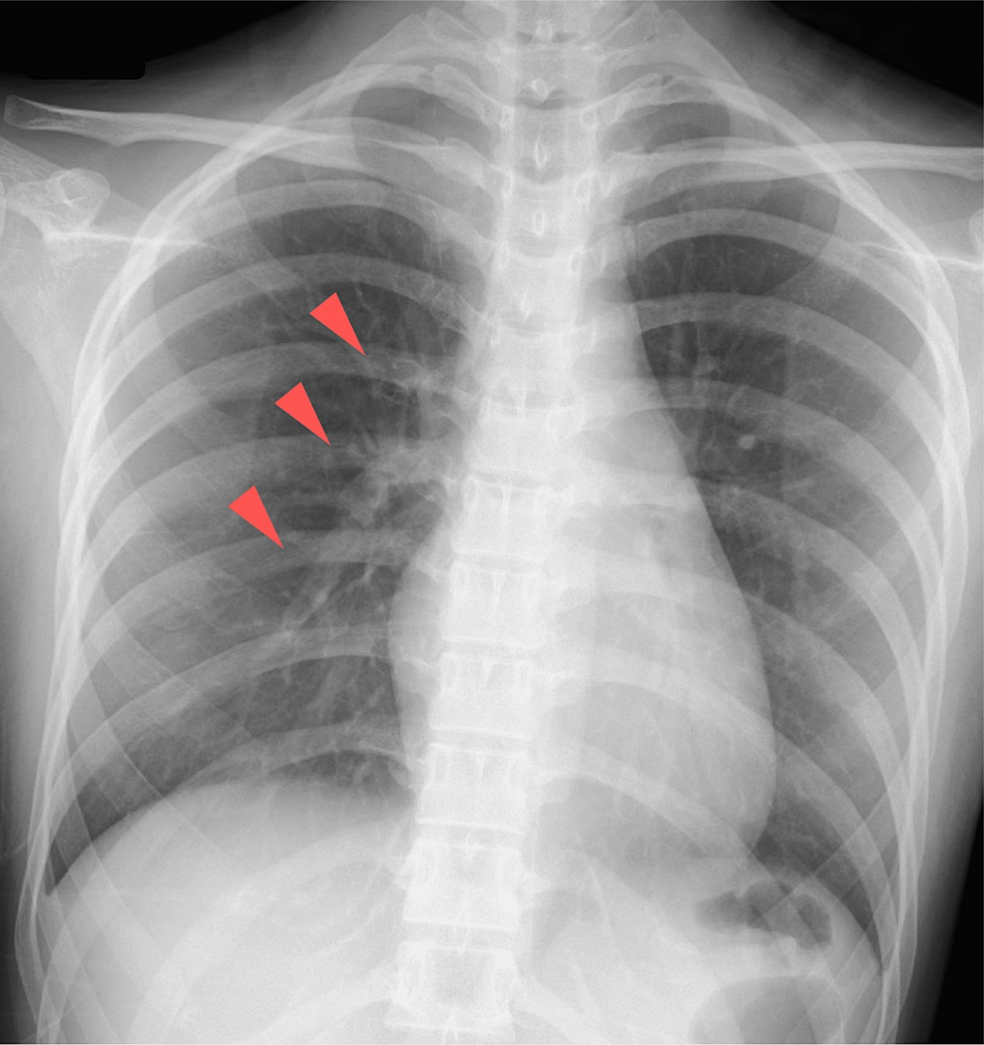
Chest X-ray on admission The chest X-ray showed a slight enhancement of hilar shadow (red arrowheads), no consolidation suggestive of pneumonia, and the cardiothoracic ratio was 45.6%.
